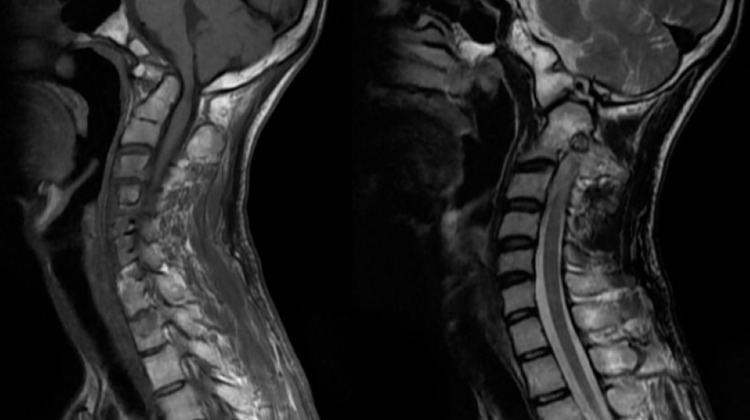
MRI cervical spine showing atlantooccipital assimilation with basilar invagination and cervical myelopathy.
